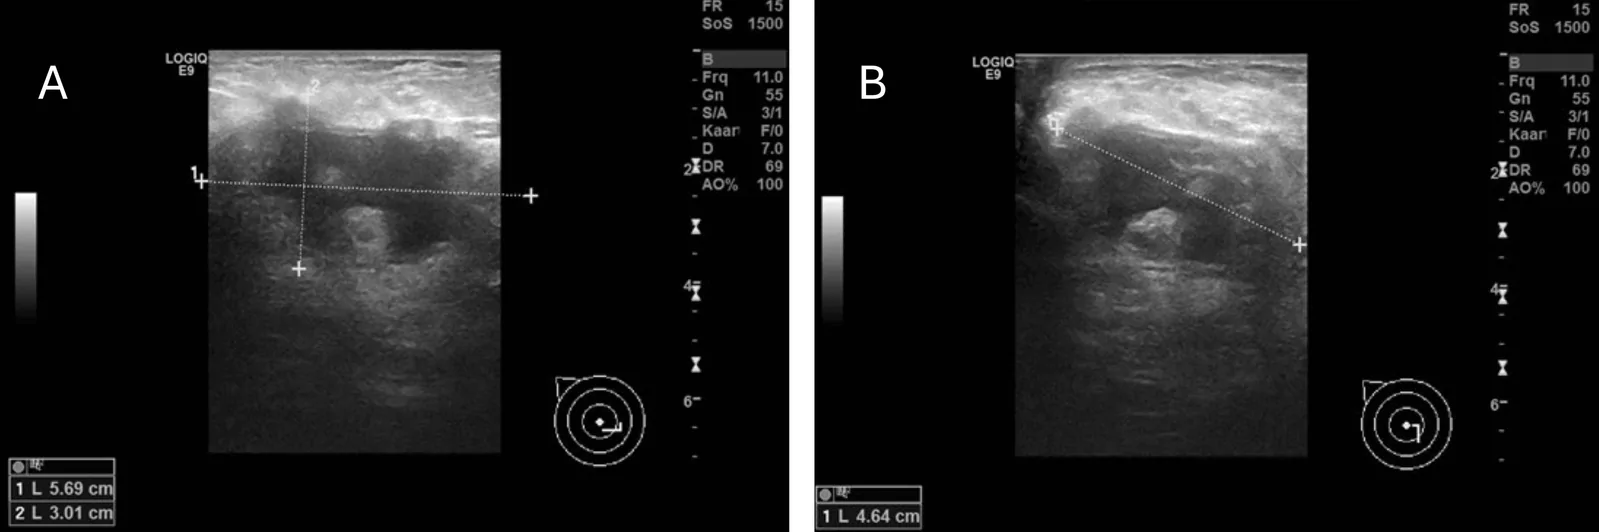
Ultrasound of the right breast revealing an ill-defined heterogeneous mass measuring 5.0 × 4.6 × 3.0 cm with posterior acoustic enhancement (A–B) (BI-RADS IV).
